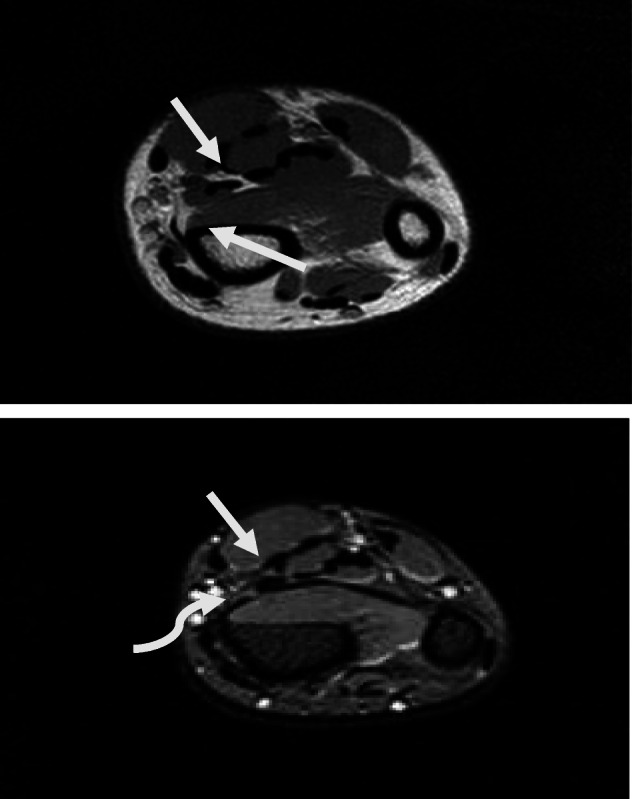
a T1 and b T2 fast field echo magnetic resonance imaging of the right distal forearm (axial scan) revealing that the mass is isointense with the muscles of the forearm (the *straight* arrow indicates the hypertrophied muscle and the *curved arrow* indicates the median nerve)
